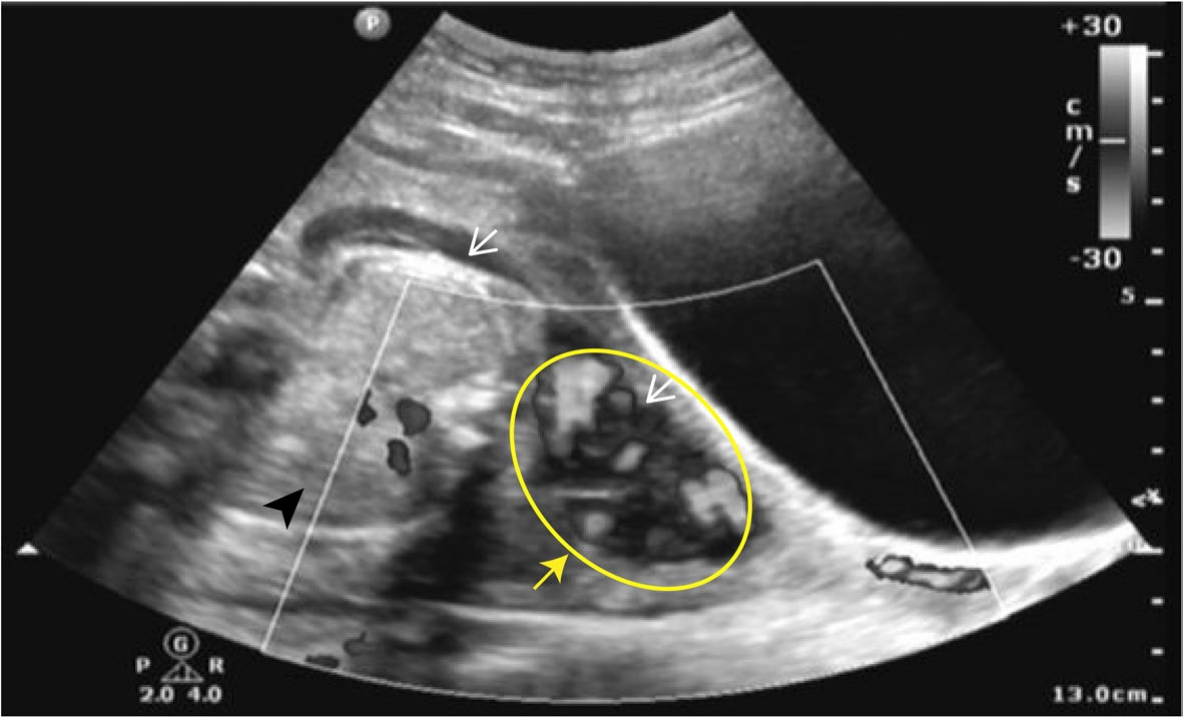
Obstetric ultrasonography after admission. There is hardly any amniotic fluid in the amniotic sac in both hemi-cavities (white arrows). The fetus curls up in the right hemicavity and the umbilical cord (yellow circle and yellow arrow) is huddled in the left side directly above the internal cervical os
